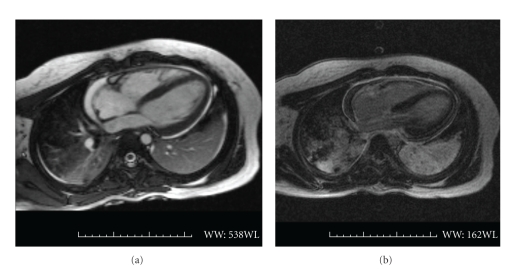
(a) Steady-state free precession cardiac MRI 4 chamber image showing small pericardial effusion, trace pleural effusion, and posterior lung field atelectasis. (b) Delayed enhancement cardiac MRI image (10 minutes postgadolinium infusion) showing no myocardial delayed enhancement; thus, no fibrosis. Visceral and parietal pericardium noted in white with pericardial effusion appearing dark between these two layers.
